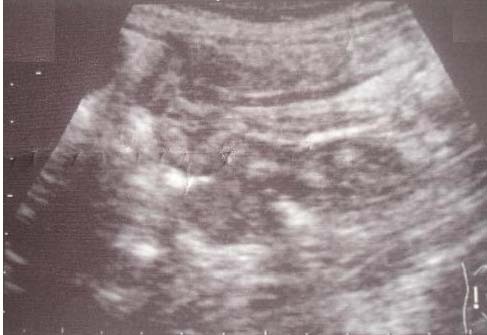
Ultrasonographic image of intussuscepted bowel in longitudinal plane "sandwich" appearance.
